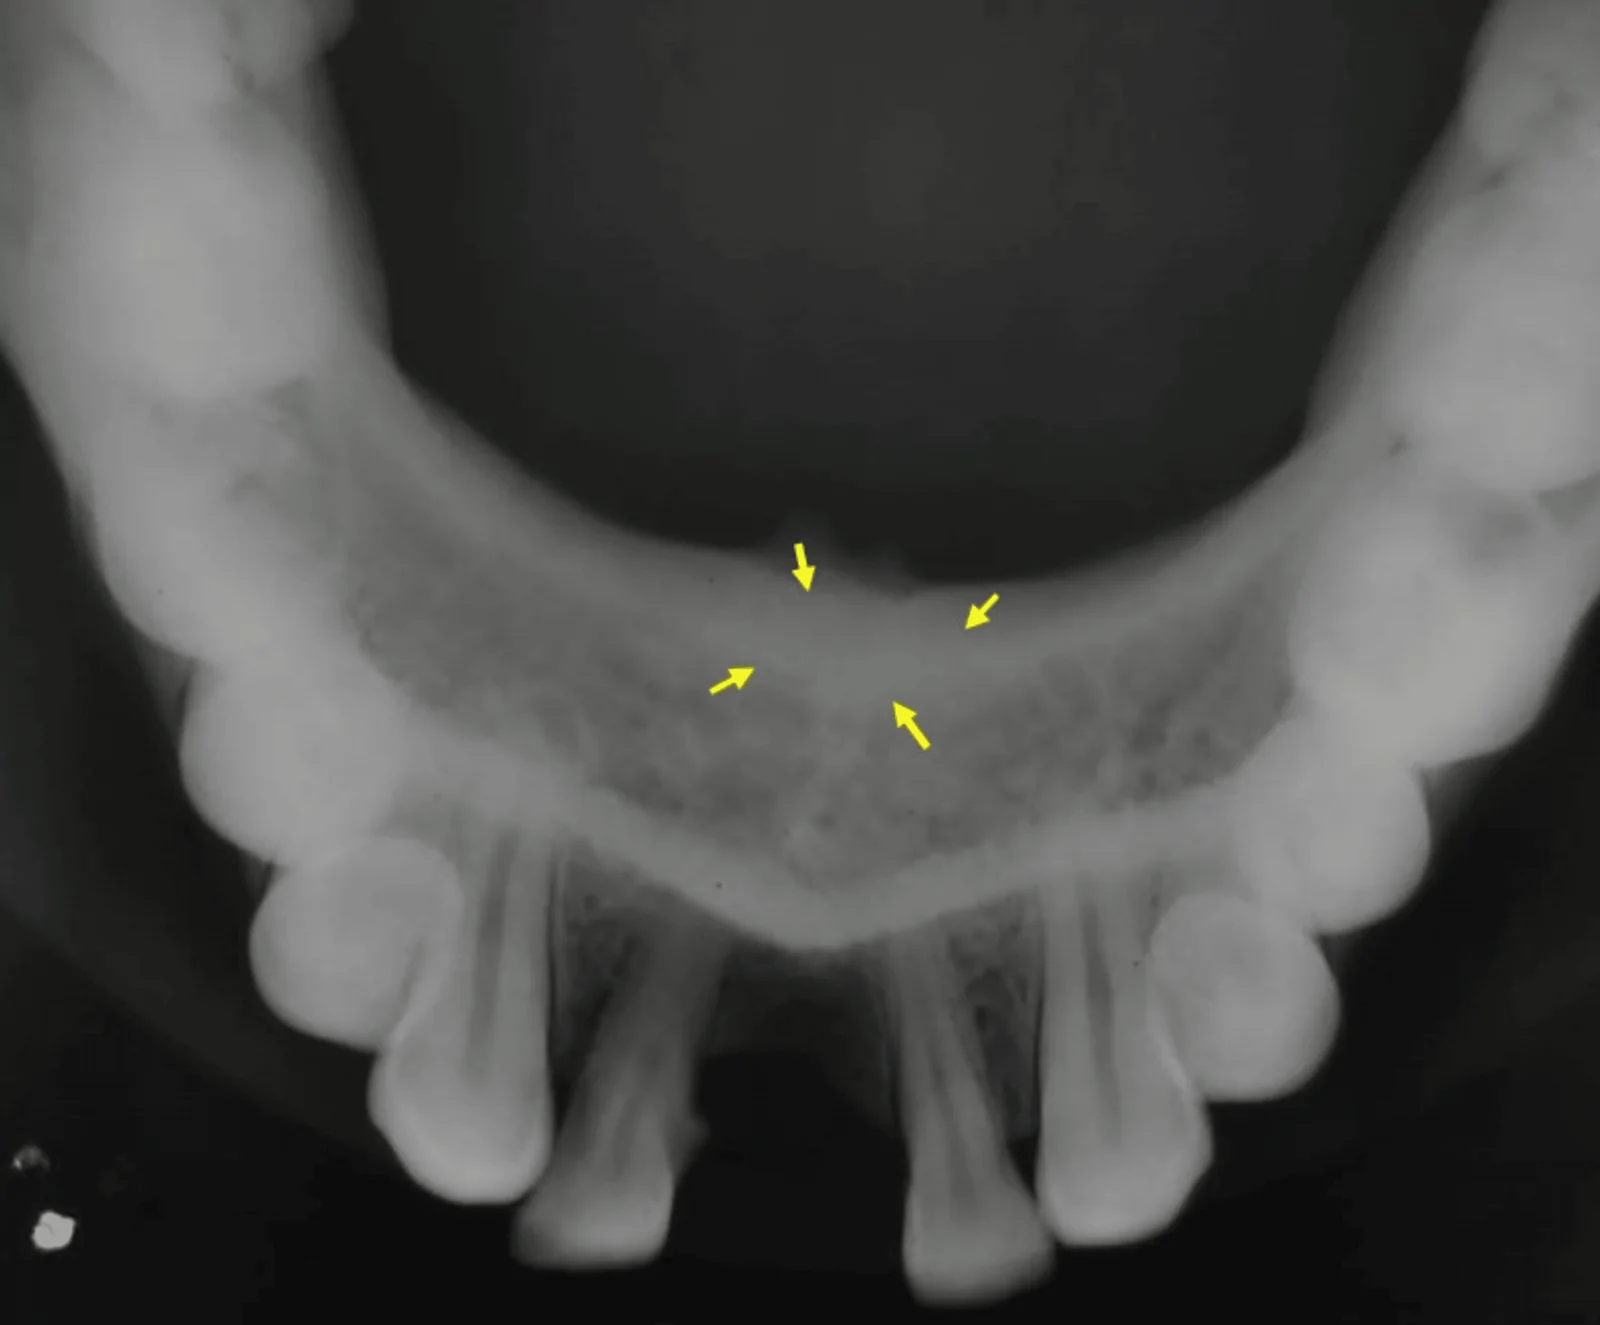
Topographic mandibular occlusal radiograph revealed a dense radiopaque mass superimposing the area of genial tubercle (yellow arrow).
